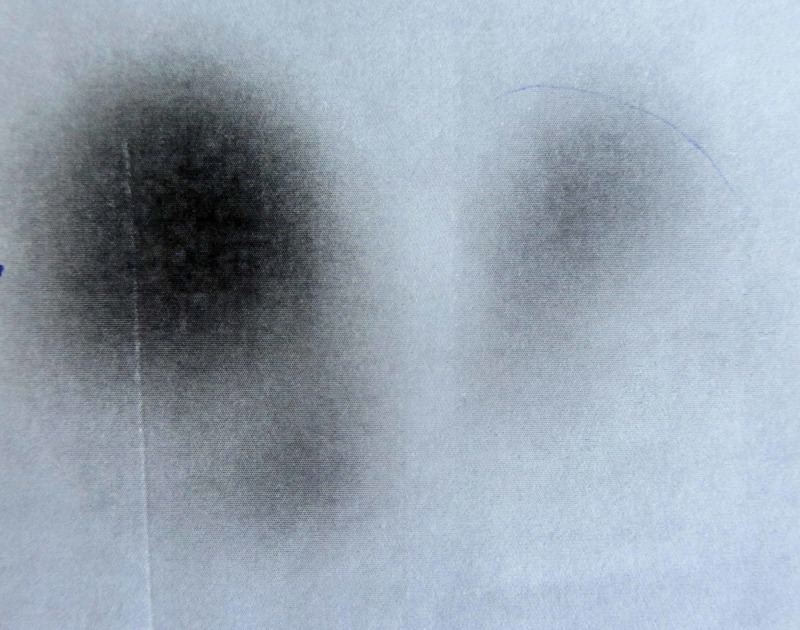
Nuclear scan of the thyroid Thyroid scintigraphy shows functioning "hot" nodule, adenoma in the right lobe.
